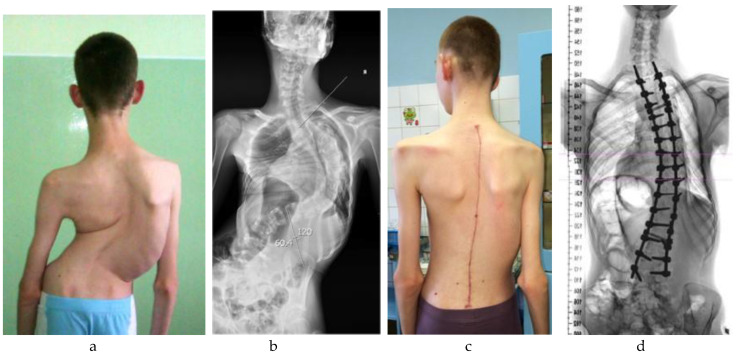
An adolescent patient with neuromuscular scoliosis secondary to cerebral palsy. In contrast to the case presented in Figure 1, this patient retained ambulatory function and did not require pelvic fixation: (a) Preoperative clinical image demonstrating moderate coronal imbalance with visible scapular asymmetry; (b) Preoperative standing anteroposterior radiograph showing a structural thoracolumbar scoliosis without pelvic obliquity; (c) Postoperative clinical image showing improved trunk alignment and a well-healed midline incision; (d) Postoperative radiograph demonstrating correction of the scoliotic curve with segmental posterior instrumentation terminating above the pelvis.
